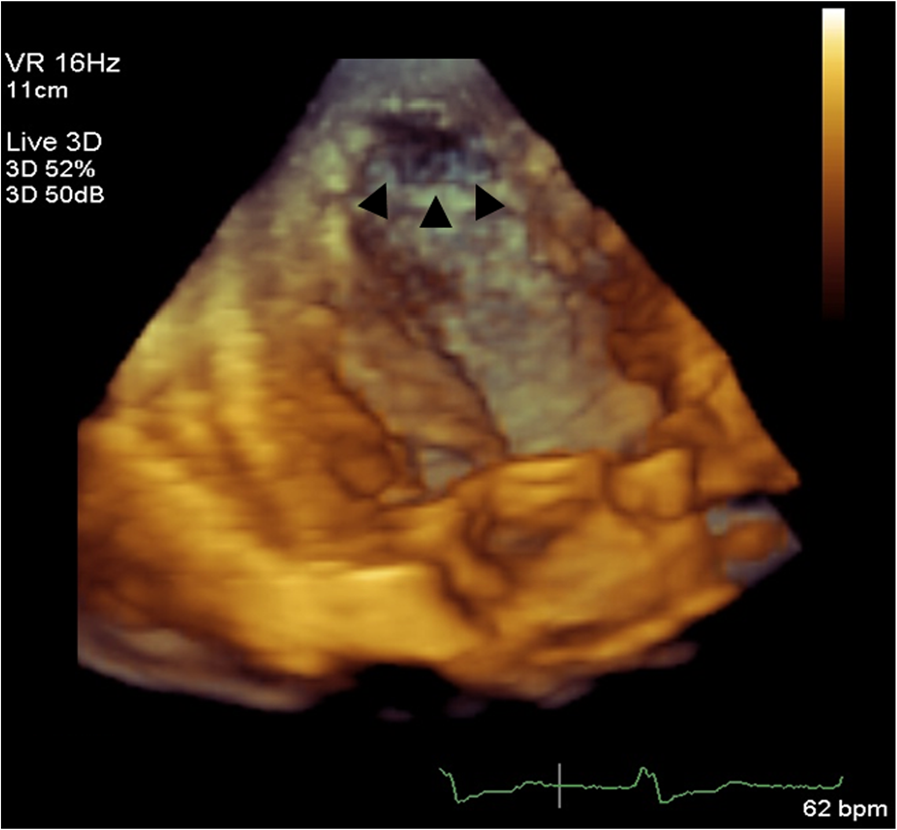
Real-time three-dimensional echocardiography demonstrates the typical ‘honeycomb appearance’ (arrowheads) in the apical lateral wall in our patient (Case 1).
